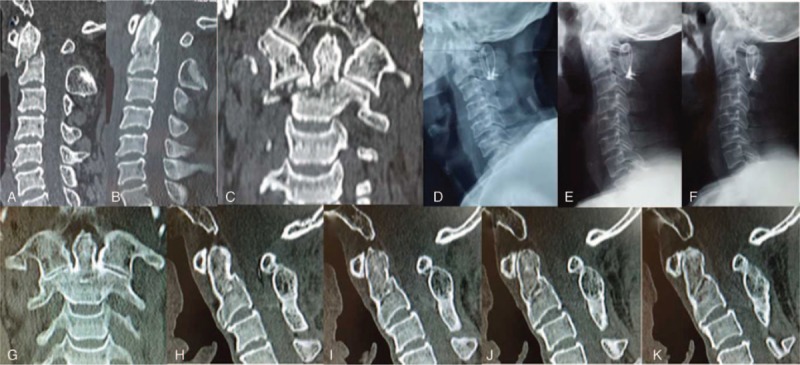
Male, 35 years old, duration from injury to operation was 5 days. (A) Preoperative CT showed type II C odontoid fracture, with fracture anterior displacement = 7.3 mm. (B, C) Reduction of the displaced odontoid fracture was attempted using the Mayfield head-holding device. (D–F) Postoperative lateral, flexion, and extension X-ray showed the cervical alignment and mobility was excellent after modified Gallie graft fusion-wiring instrumentation. (G–K) Twelve-month follow-up CT scan showed evident bridging bone across the odontoid fracture site in coronal and sagittal CT-scans in neutral head position.
